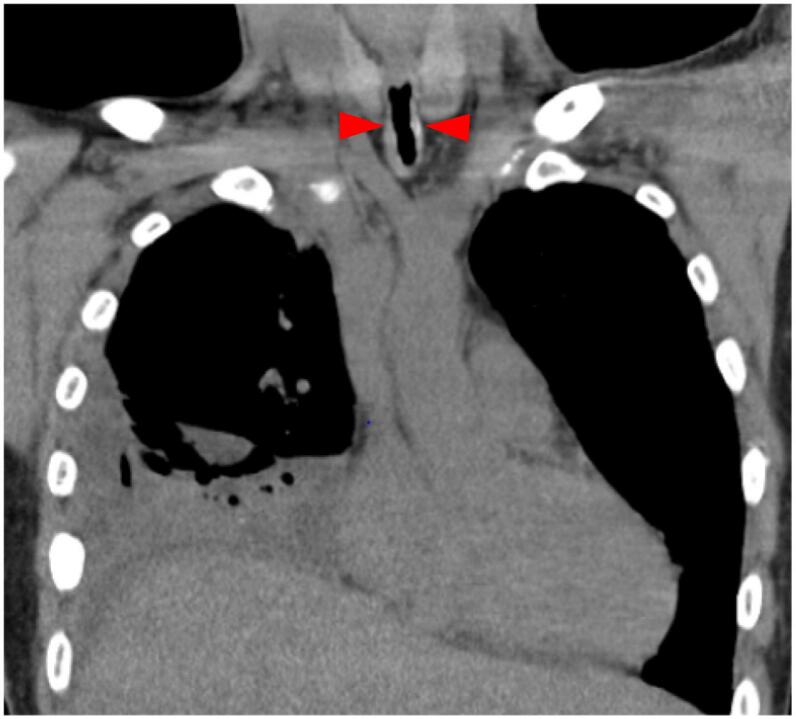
Chest CT of the narrowest area of trachea. The diameter of tracheal narrowing area was 6 mm at preoperative CT.
